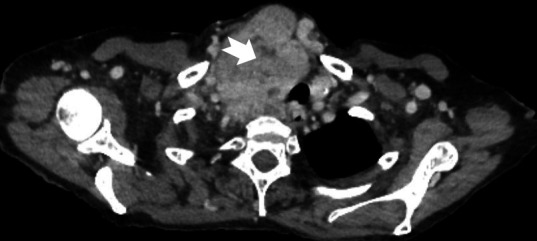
Axial CT image at the level of T3.
